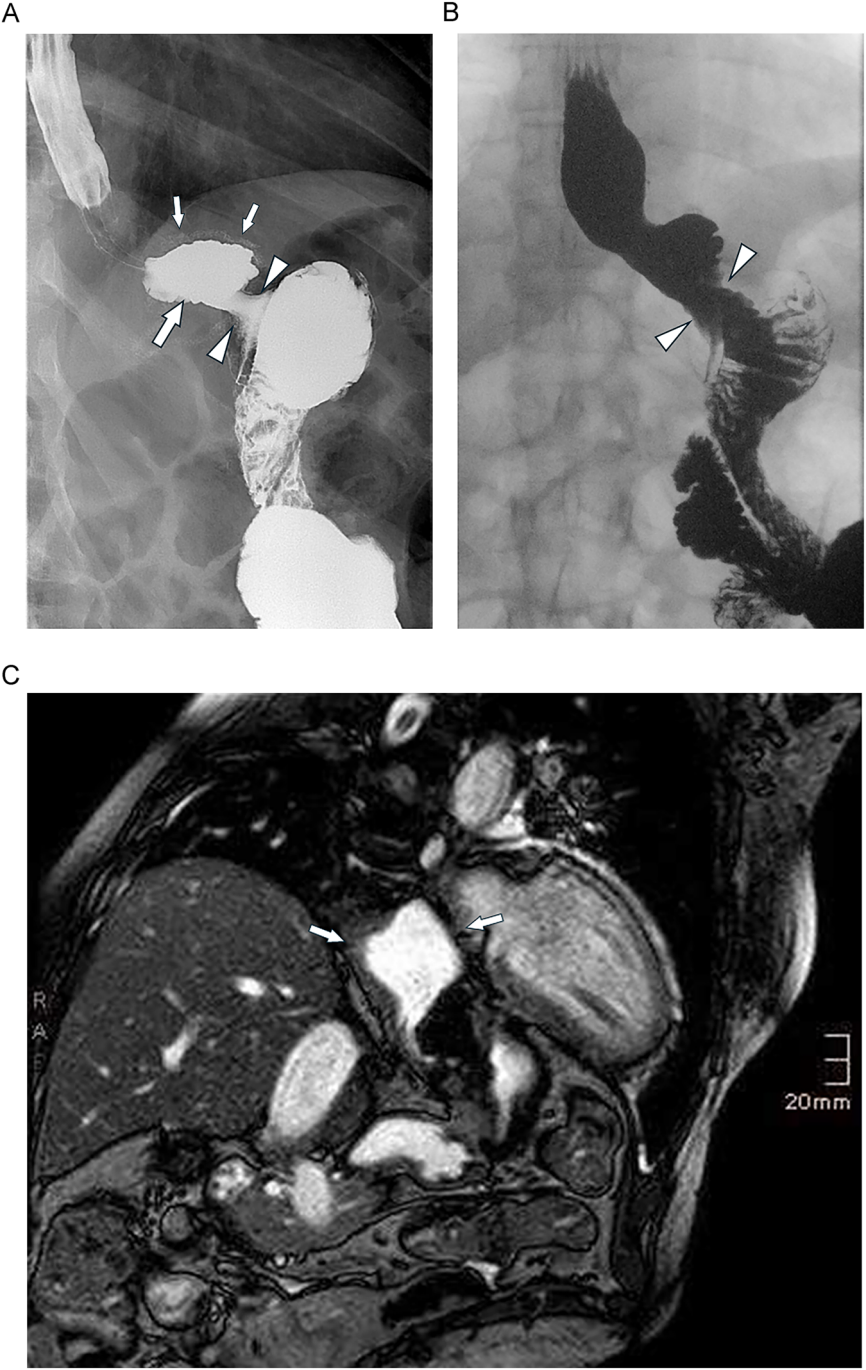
Normal postoperative anatomy at an upper GI study. A The gastric pouch is demonstrated (large arrow) with surgical staples along the contour (small arrows). The width of the gastro-jejunostomy anastomosis (arrowheads) cannot be assessed on this static image. B The cine sequence during swallowing shows normal widening of the anastomosis (arrowheads). C Dynamic MRI using a steady-state free precession sequence (True-FISP) shows the size and position of the pouch (arrows) after administration of oral contrast
